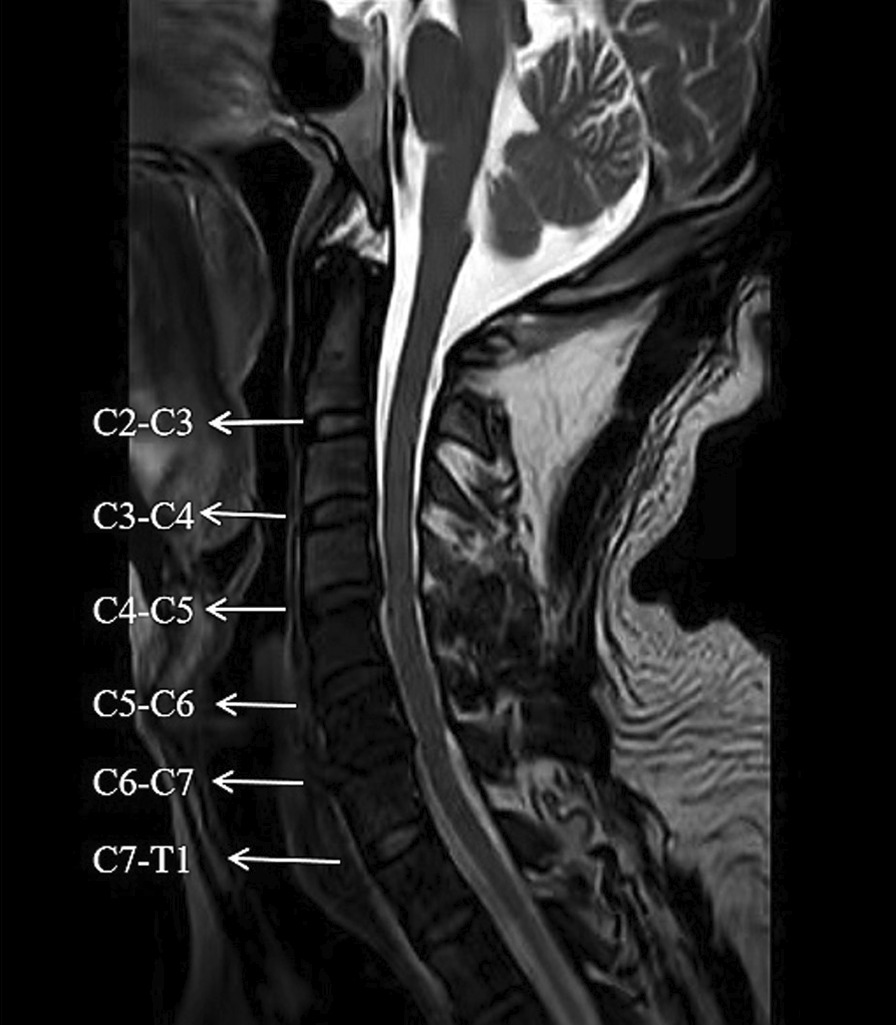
Sagittal T2‐weighted cervical MR imaging of a patient. Pfirrmann classifications of the cervical discs were as follows: C2–3 was Grade 2, C3–4 was Grade 3, C4–5 was Grade 3, C5–6 was 4, C6–7 was Grade 5, and C7–T1 was Grade 2. The total Pfirrmann scores of the patient were 19. Likewise, the total Miyazaki scores = 1 + 2 + 3 + 3 + 4 + 1 = 14, total modified Thompson scores = 0 + 1 + 2 + 1 + 3 + 0 = 7, and the total Nakashima scores = 1 + 1 + 1 + 2 + 3 + 1 = 9
